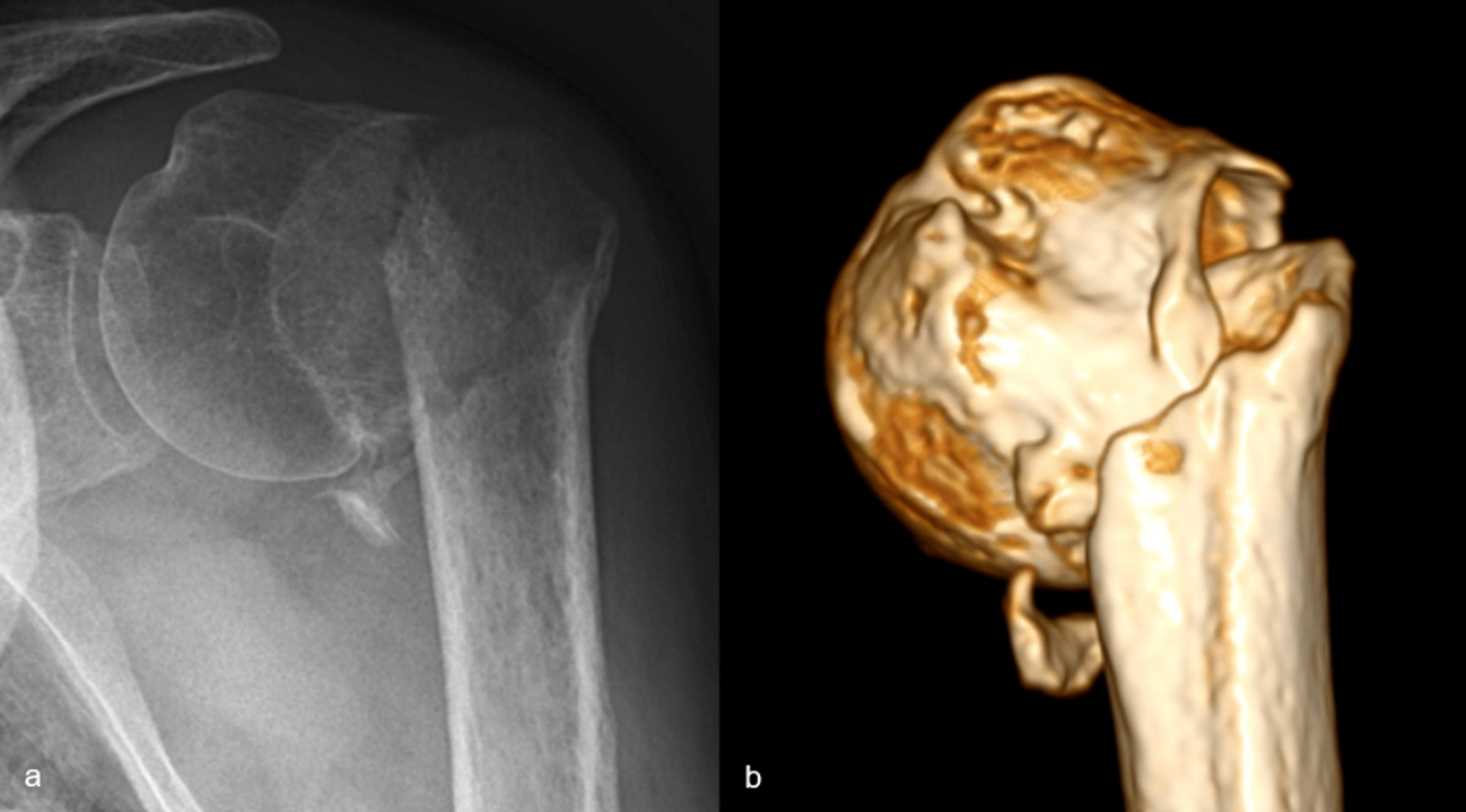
AP X-ray (a) and 3D-CT image reconstruction (b), seven months after the initial trauma: established non-union in about 80° varus position, with excavation of the humeral head, but good cortical bone on both sides CT: computed tomography
